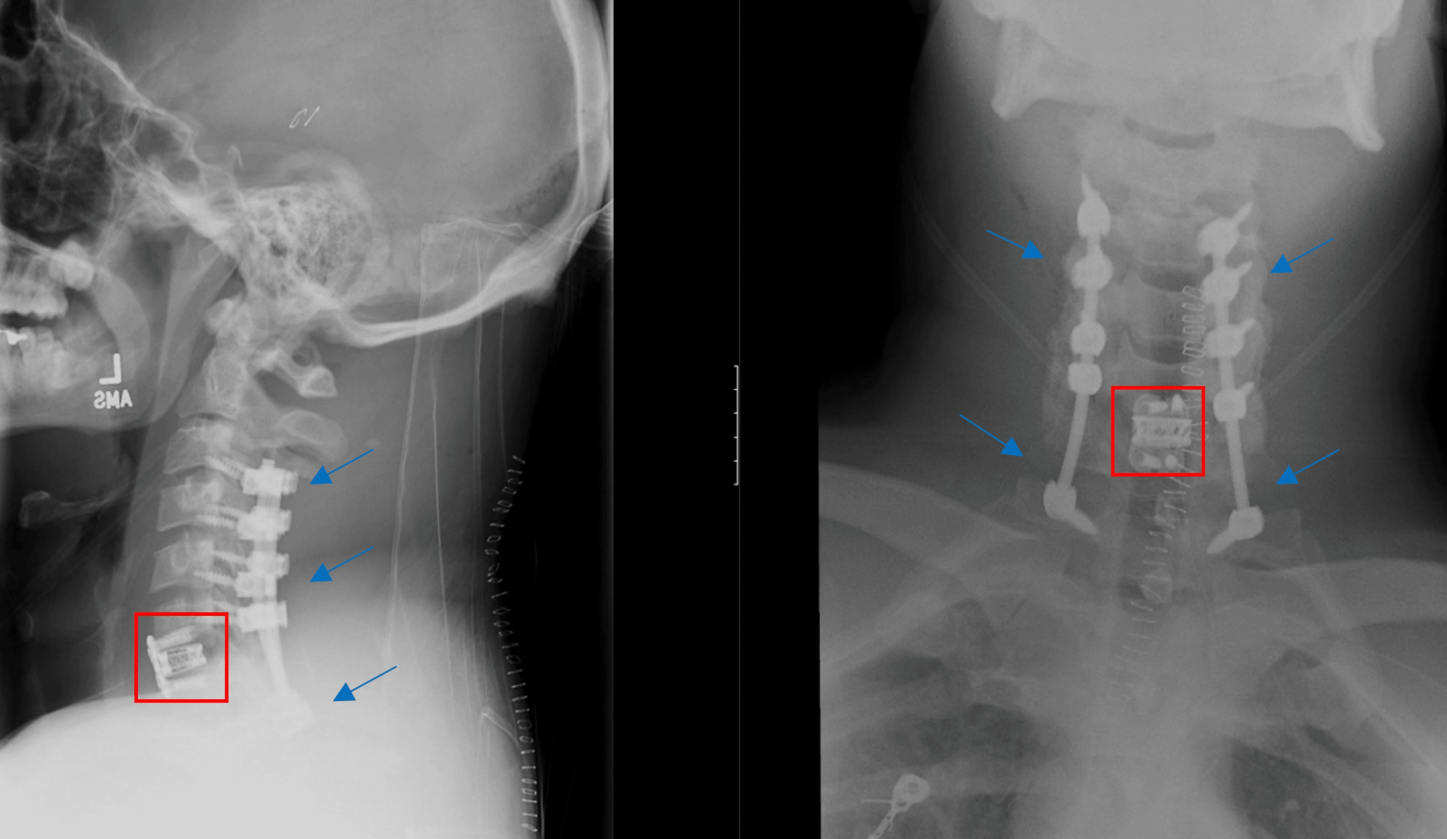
Lateral (left) and anterior-posterior (right) plain films of surgical construct, showing C6-7 anterior cervical discectomy and fusion (red box) with C3-T1 posterior cervical decompression and fusion (blue arrows).
